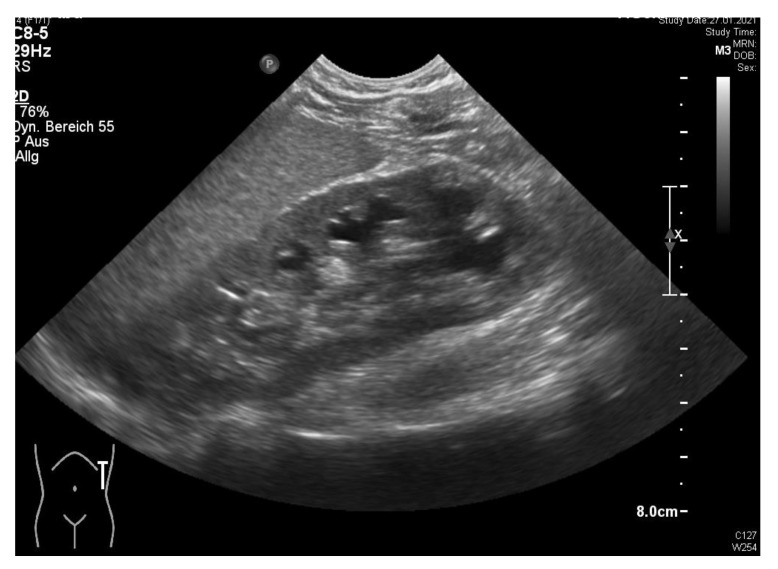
Ultrasound test after left-sided robotic pyeloplasty before dismission shows normal kidney without dilatation of the pyelon (courtesy of Dr. A. Longin, Klinikum Esslingen).
